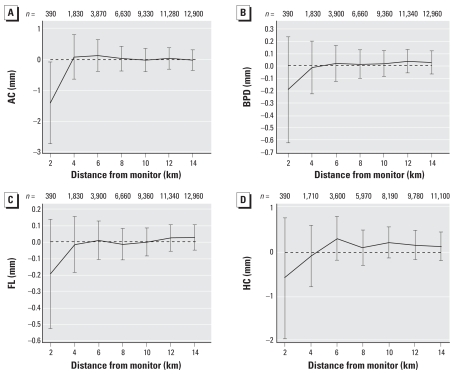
Change in (mm) in ultrasound measurements for an 8-ppb increase in O_3_ during days 31–60 of gestation by distance to monitor. (*A*) AC; (*B*) BPD; (*C*) FL; (*D*) HC.
